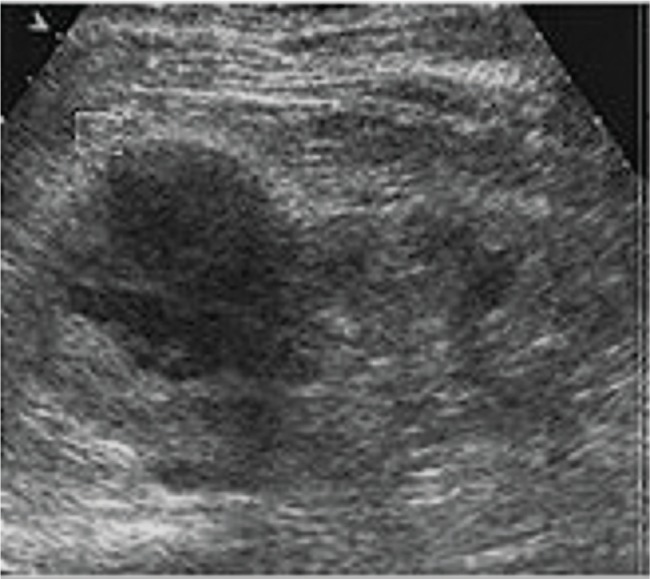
Grayscale ultrasonography showing a suspected mass-mimicking lesion in the right kidney in a 53-year-old man with clear cell renal carcinoma.
